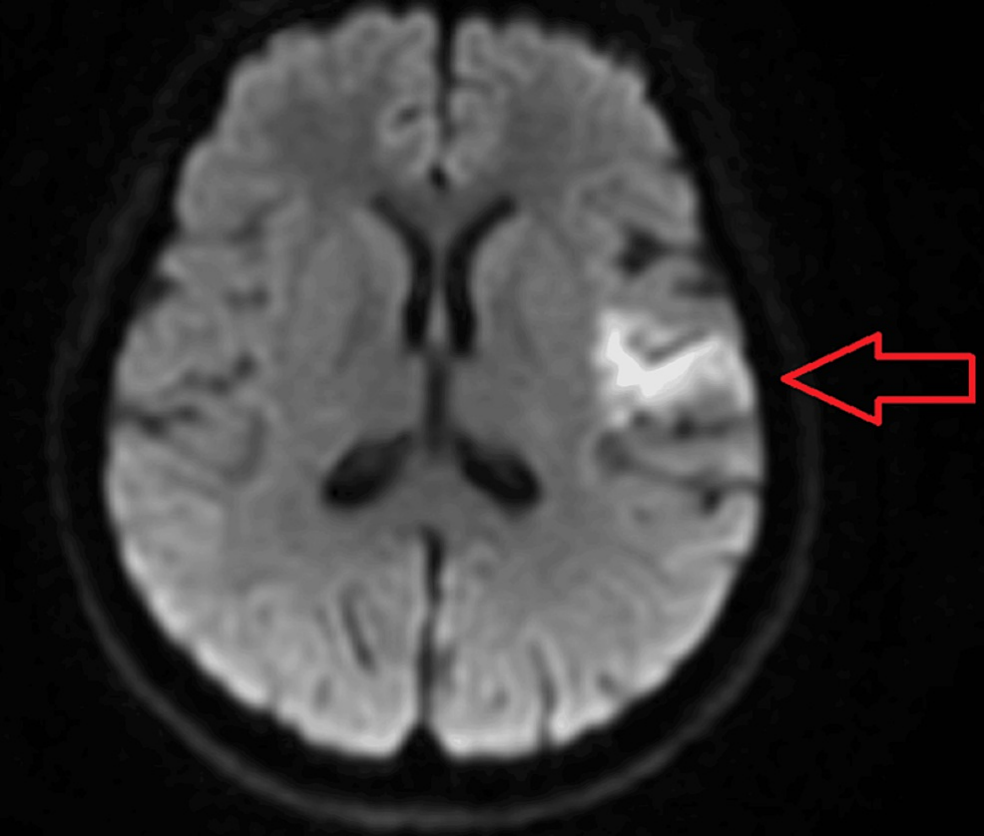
Diffusion-weighted imaging (DWI) sequence of the MRI showing acute infarction in the left frontoparietal region and insular cortex.
